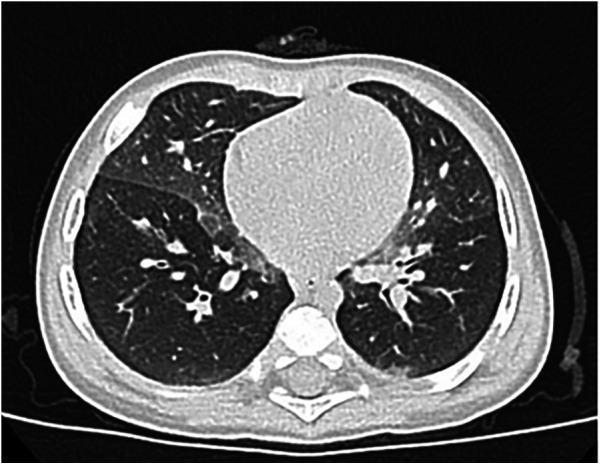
Chest HRCT: heterogeneous pulmonary density with a “mosaic” pattern, characterized by the presence of confluent “ground-glass” areas, suggestive of alveolar interstitial disease.
